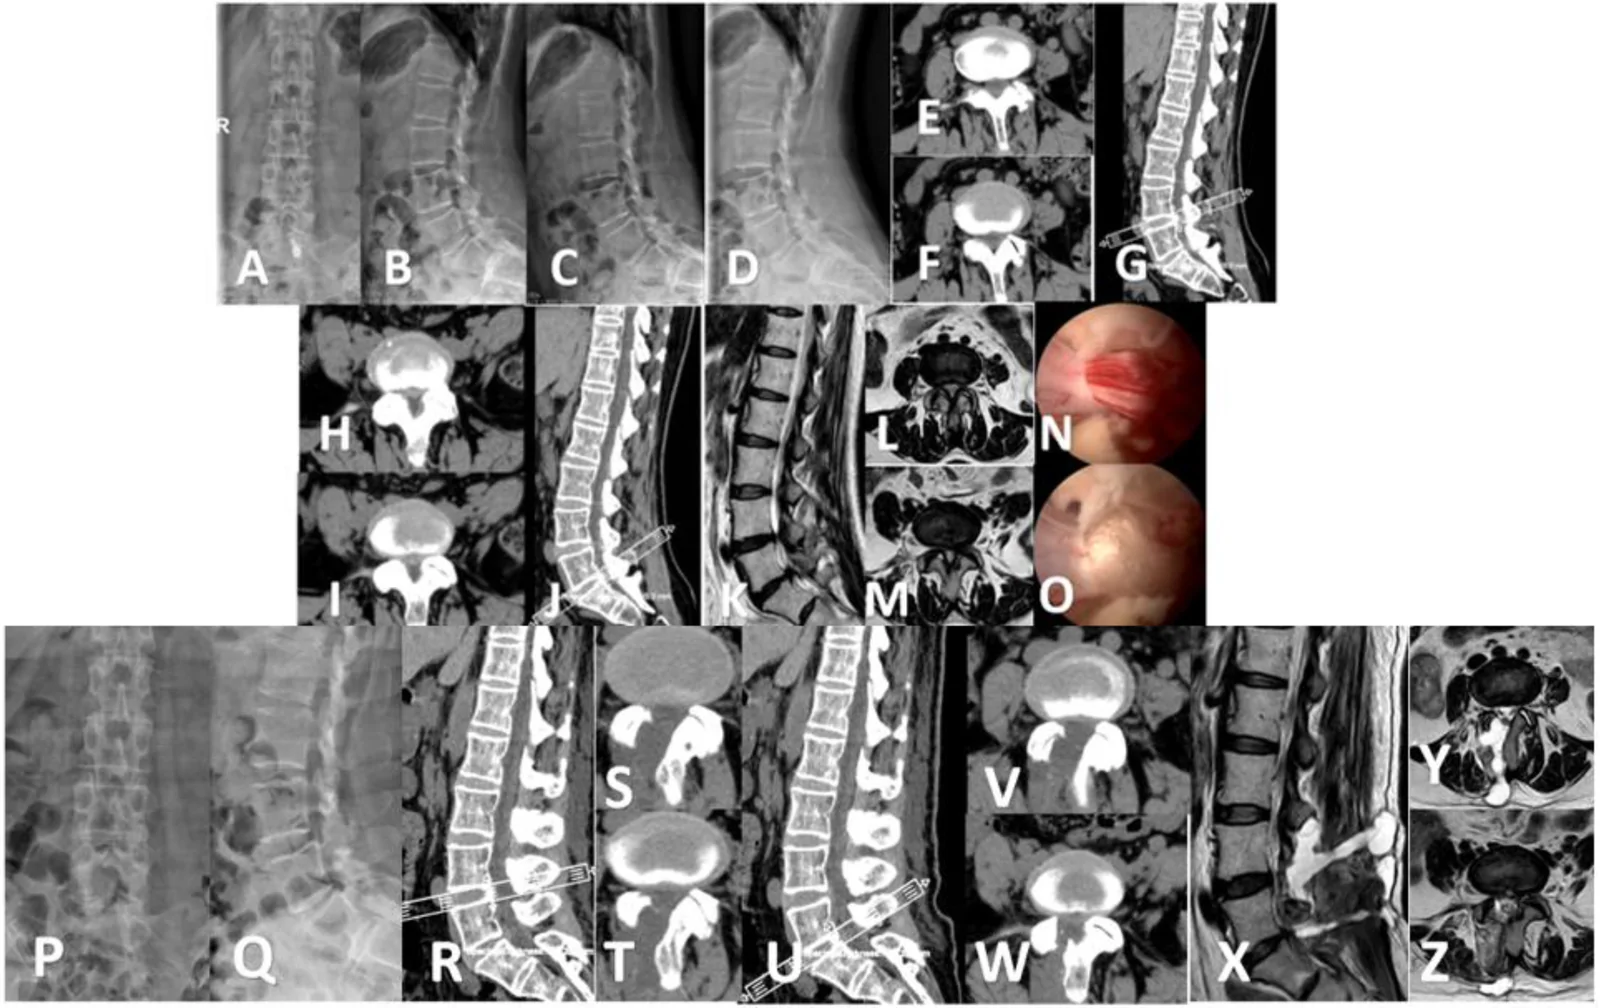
(A–D) preoperative lumbar spine anteroposterior, lateral, and flexion-extension views showed no significant instability. (E–J) Preoperative lumbar spine CT demonstrated lumbar spinal stenosis at L4–S1 without osseous protrusion. (K–M) Preoperative MRI revealed severe stenosis and soft disc protrusion at L4–S1. (N,O) Intraoperatively, dural tear was observed and was packed with gelatin sponge. (P,Q) Postoperative lumbar spine anteroposterior and lateral views showed no evidence of instability. (R–Z) Postoperative CT and MRI demonstrated adequate decompression with evidence of cerebrospinal fluid leakage.
